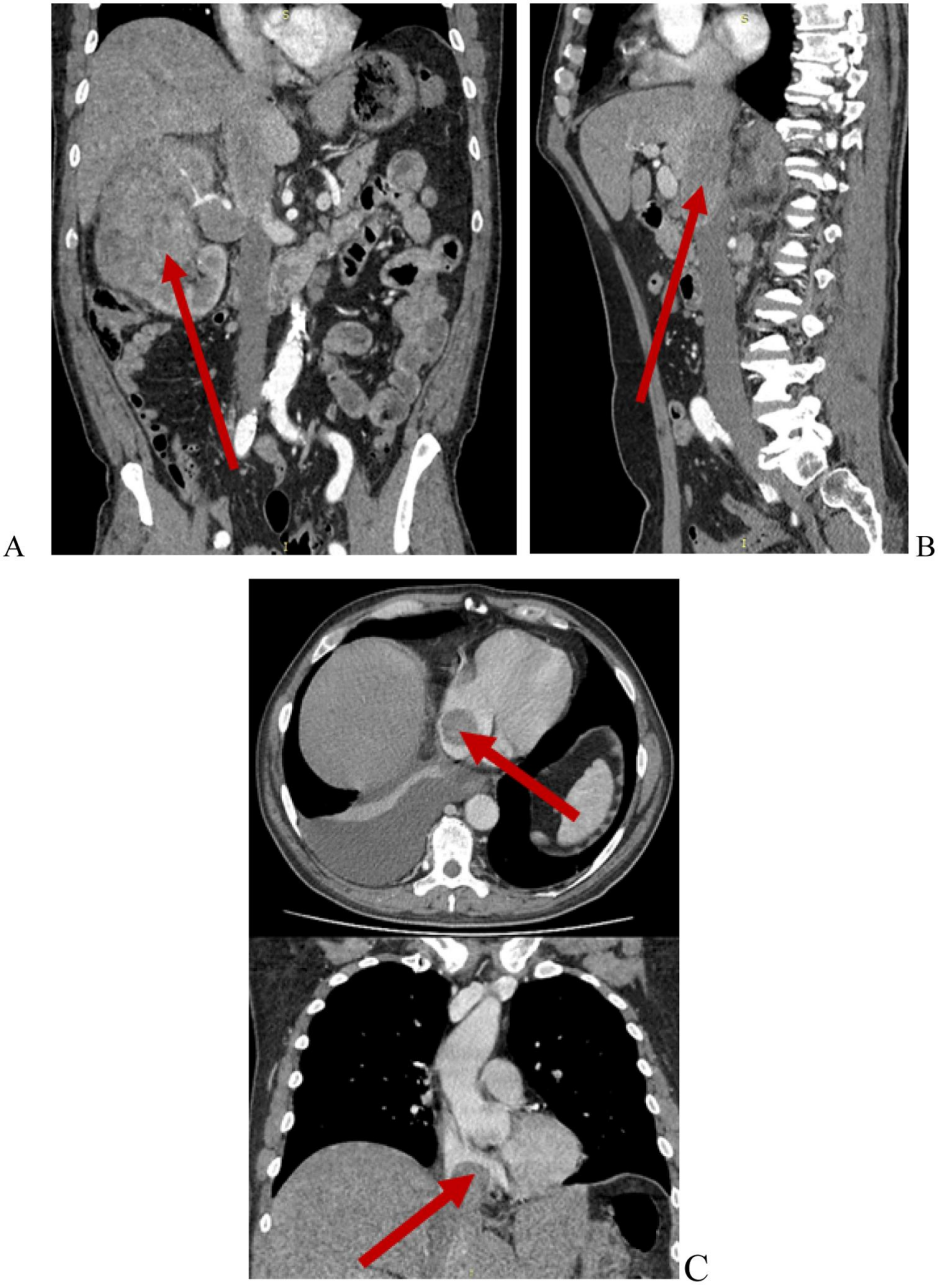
Contrast-enhanced abdominal CT. Renal cell carcinoma with Mayo level IV IVC tumor thrombus. (A) Renal tumor (red arrow); (B) Tumor thrombus within the IVC and right hepatic vein (red arrow); (C) Tumor thrombus extending into the right atrium (red arrows).
